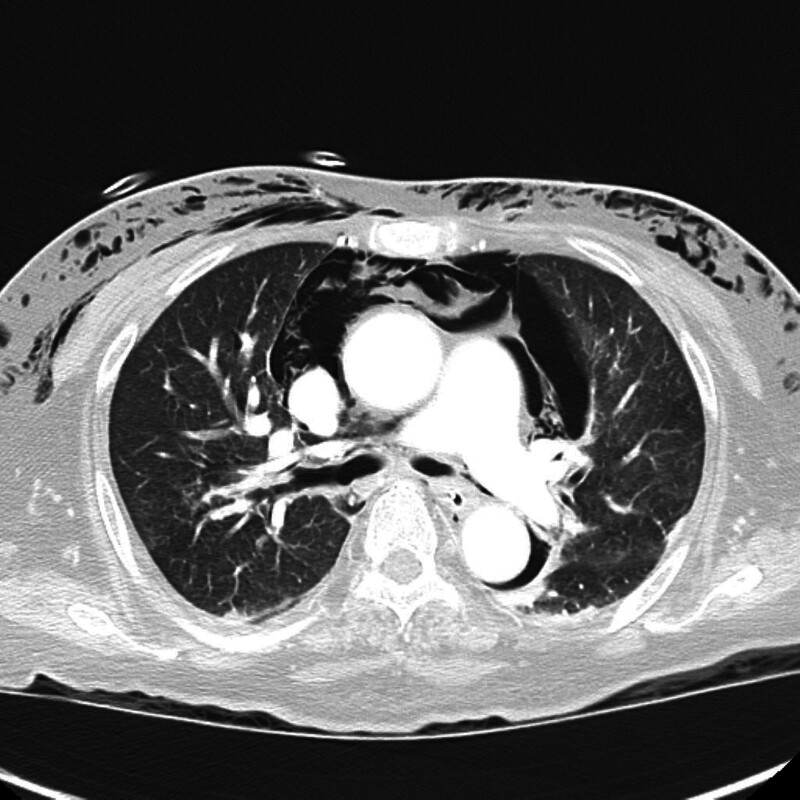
Axial chest CT at the mid-thoracic level reveals moderate bilateral pneumothorax. Both lungs show peripheral lucency, indicating free air in the pleural space, more prominent on the left side. The partially collapsed lungs exhibit peripheral atelectasis, and air density is visible in the subcutaneous tissues along the chest wall, indicating ongoing subcutaneous emphysema. CT = computed tomography.
